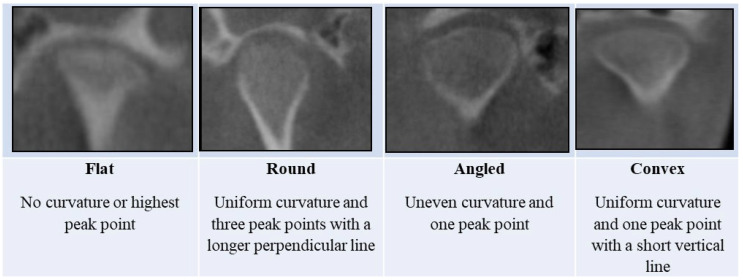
Proposed CBCT classification for the mandibular condylar shape.
